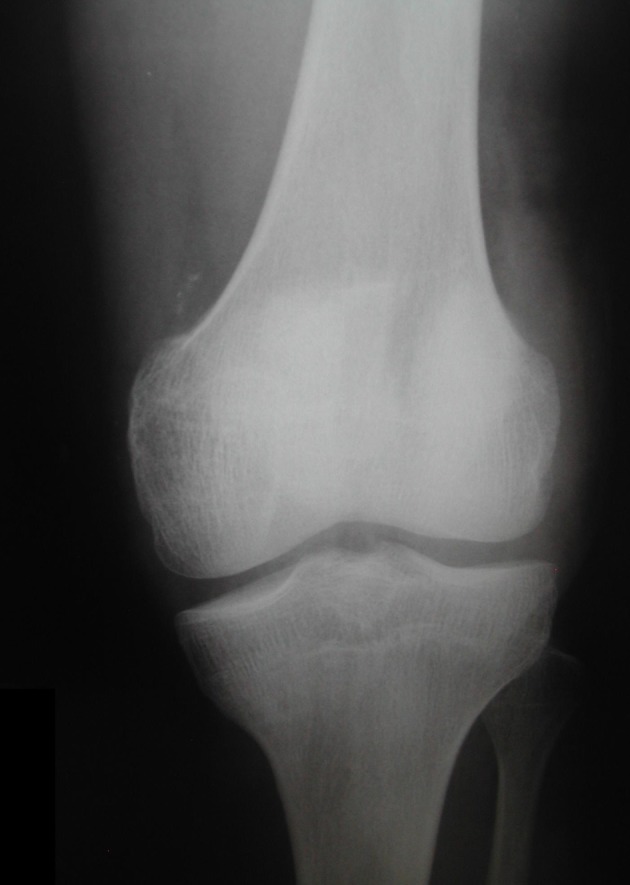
Anteroposterior radiograph demonstrated a vertical displaced patella fracture. Note that the deviated fragments are separated more than 3 mm.
